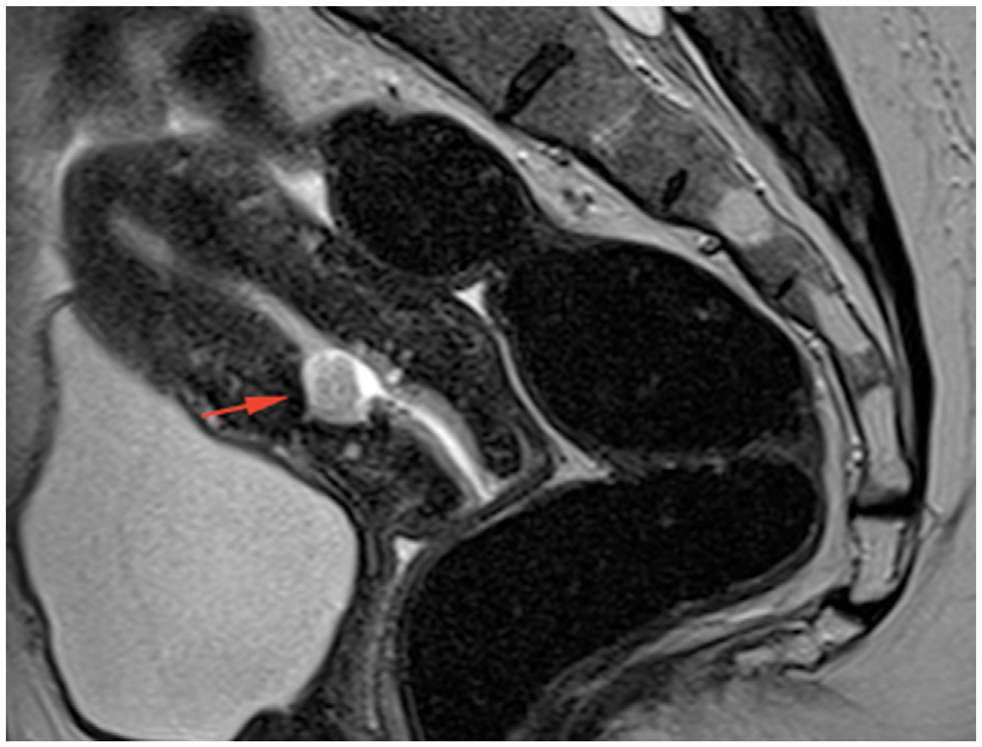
Sagittal T2-weighted TSE MRI image 1 month after medical treatment showing a small residual cavity containing some debris at the site of the abscess.
